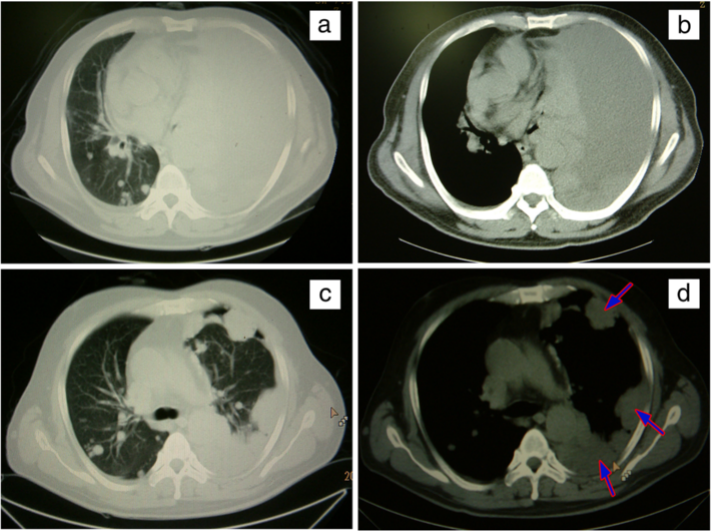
The chest CT imaging of the pleural effusion and lesions. Before operation, pleural effusion and lesions in the left thorax and multiple metastases in the right lung can be seen in CT scan imaging (a and b). After complete drainage of pleural effusion, metastatic neoplasms in pleura, enlargement of mediastinal lymph nodes, and lung metastases were clearly emerged by countercheck CT scan (c and d). The pleural lesions were pointed by *blue arrows* in d. a and c Lung window. b and d Mediastinum window
